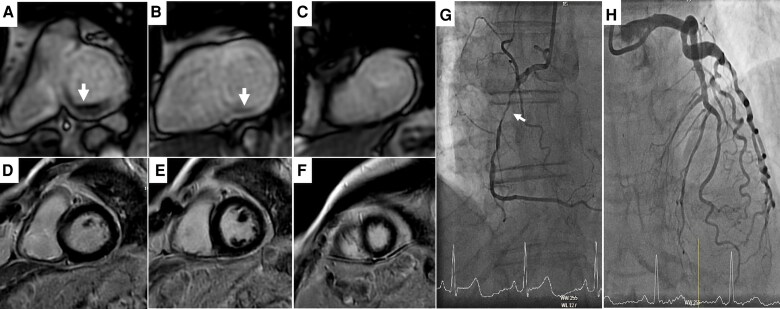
Stress cardiovascular magnetic resonance imaging in a 68-year-old male patient with atypical chest pain and a history of lung carcinoma treated with chemotherapy and radiotherapy. Perfusion images (*A*, *B*, *C*) acquired after the administration of adenosine demonstrate a large perfusion defect in the basal inferior wall and basal inferior interventricular septum (*A*), as well as a smaller defect in the middle portion of the inferior wall. Late gadolinium enhancement images (*D*, E, *F*) reveal no evidence of prior myocardial infarction. Invasive coronary angiography (*G*) showed subtotal occlusion of the mid-right coronary artery (arrow).
